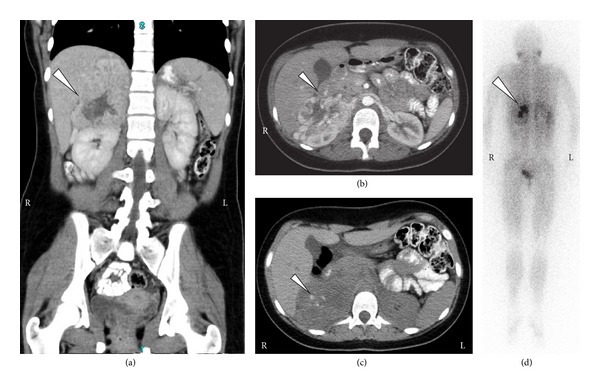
Radiologic imaging of the patient at presentation. (a, b, c) Computed tomography images showing a right-sided contrast-enhancing suprarenal mass (white arrows) displacing both the kidney and the liver. Note calcifications in the mass (arrow; image (c)), which is typical for pheochromocytoma. (d) MIBG nuclear scan showing uptake by the adrenal mass (arrow) and no evidence of other sites of disease.
